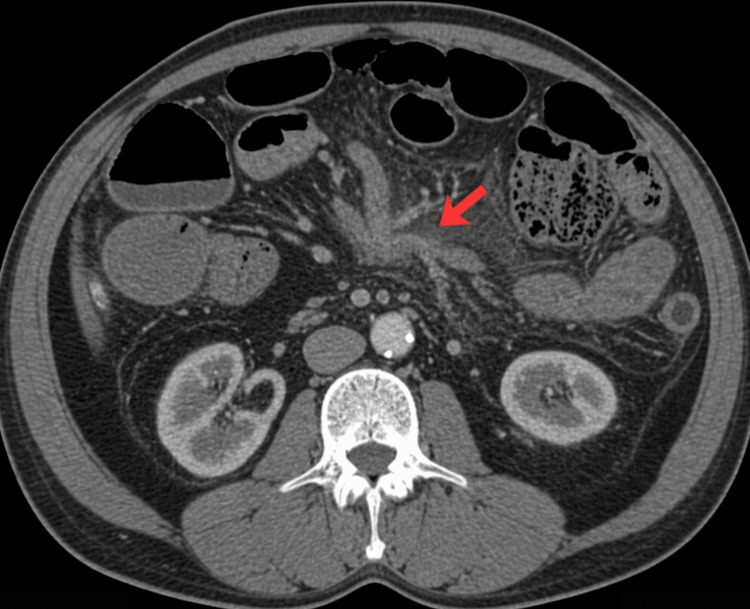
Abdominal CT scan showing mesenteric infiltration
